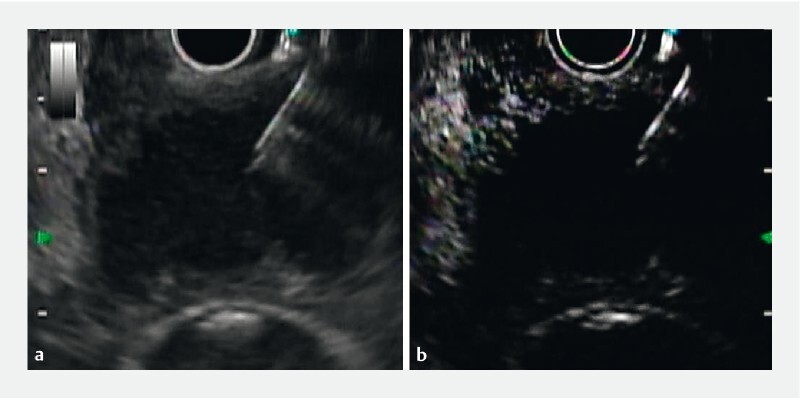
Endoscopic ultrasonography shows the fluid space of the abscess, which was punctured using a 19-gauge needle.
